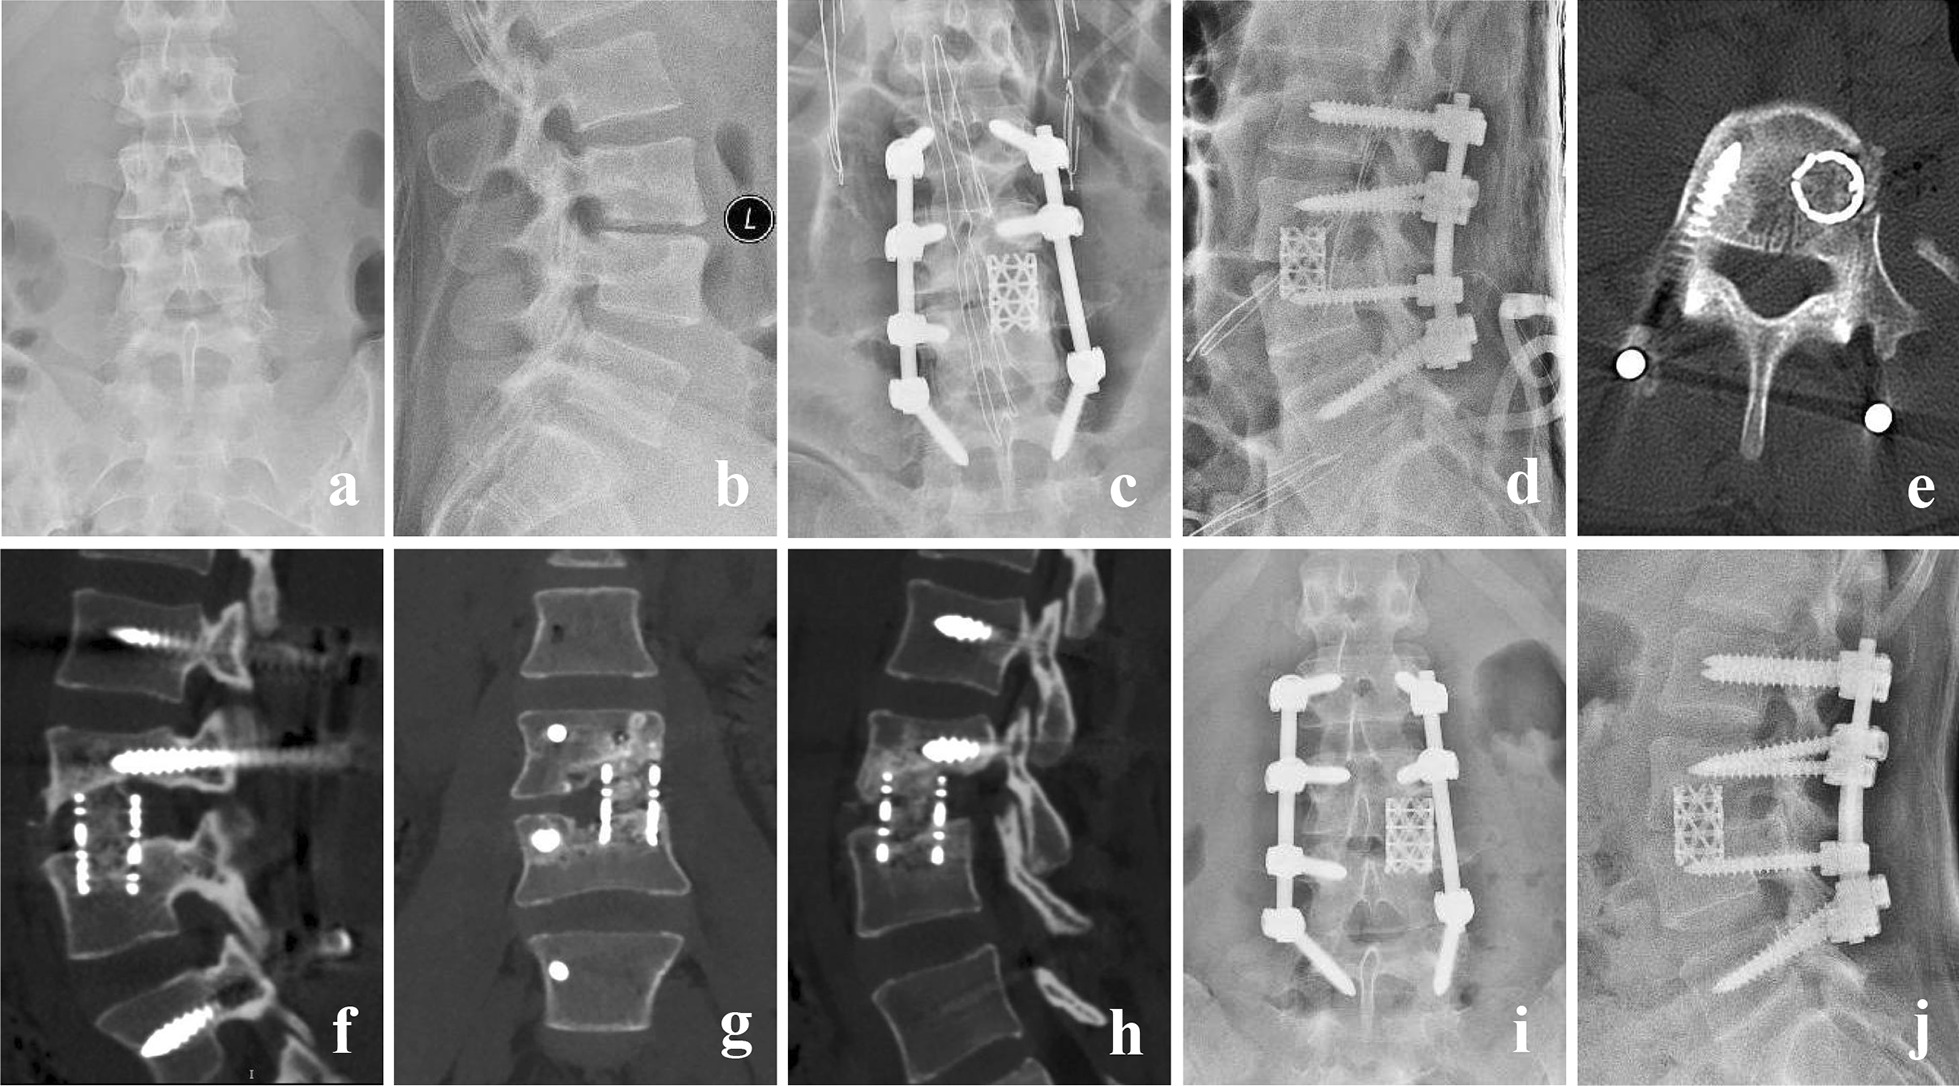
A 28-year-old female with L3-4 TB. (a, b) Preoperative X-ray showed that the vertebral gap between L3 and L4 was narrow, and vertebra body was partly destroyed. (c, d) Postoperative X-ray. (e, f) CT at 3 months postoperative showed good location of titanium cage and posterior instrument. (g, h) CT at 6 months postoperative showed bone fusion between L3 and L4. (i, j) X-ray at 14 months postoperative showed good location of titanium cage and posterior instrument
